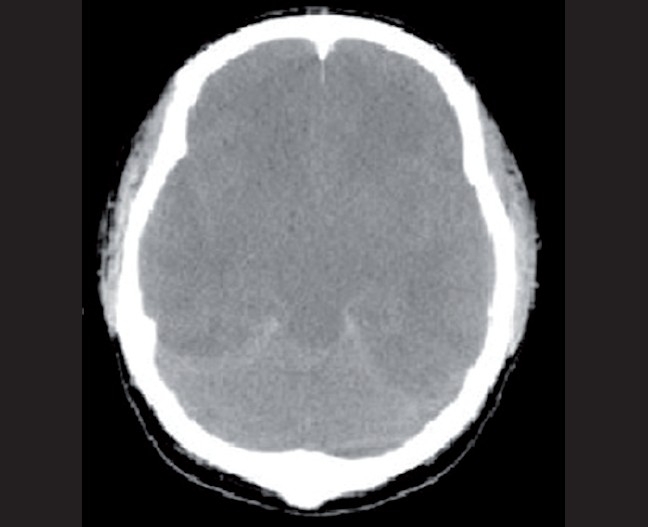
Computed tomography of the head of case 1
